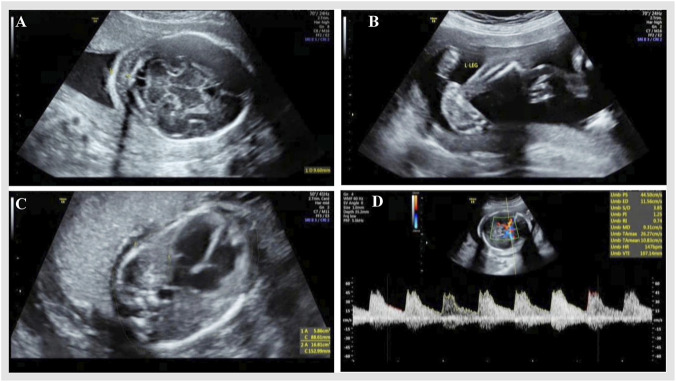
Phenotypes of the patients (A–D) Ultrasound images at 23 weeks’ gestation showing: (A) increased nuchal fold thickness (9.8 mm); (B) left clubfoot;(C) cardiomegaly with a cardiothoracic ratio of 0.35; (D) elevated middle cerebral artery peak systolic velocity (MCA-PSV: 44.5 cm/s).
